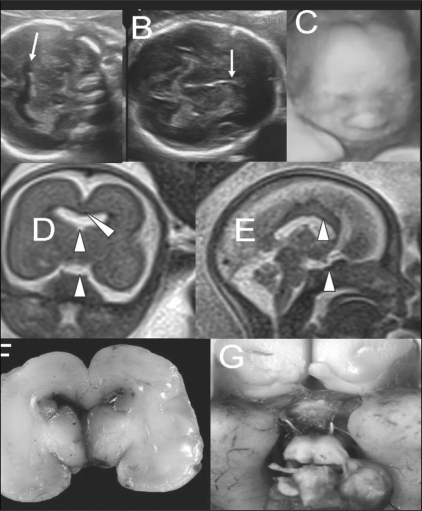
Pregestational diabetic mother with a 20 weeks' single live gestation. USG images (A,B) show fusion of the frontal horns of the lateral ventricles (arrow in A) and absent cavum septum pellucidum (arrow in B), indicating callosal agenesis. 3-D USG image (C) shows a normal fetal face. T2W coronal MRI image (D) shows absent septum pellucidum, midline cerebral fusion (solid arrowhead), and indistinct optic chiasm (open arrowhead). T2W sagittal MRI image shows the presence of a corpus callosum (open arrowhead) but a possibly hypoplastic hypophysis (solid arrowhead). Autopsy (F,G) images show frontal horn fusion, absent septum pellucidum, and a small optic chiasm, confirming the diagnosis of septo-optic dysplasia
